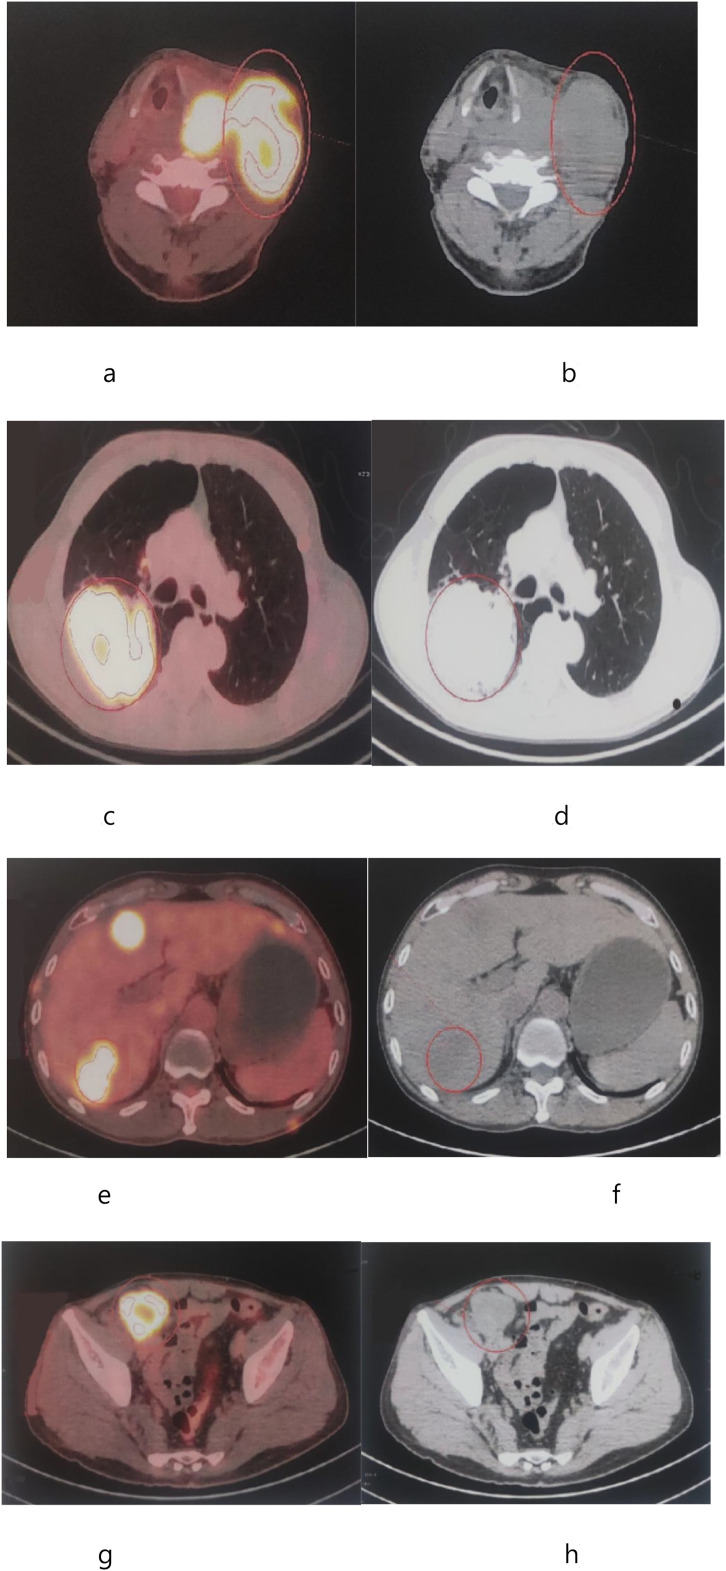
PET/CT images of the patient before treatment. (a, b) PET imaging shows increased [18F]-FDG uptake in the left thyroid (SUVmax 25.8) and lymph node (SUVmax 30). (c, d) PET imaging shows increased [18F]-FDG uptake in the right lung and pleura (SUVmax 20.6). (e, f) PET imaging shows increased [18F]-FDG uptake in the liver (SUVmax 30). (g, h) PET imaging shows increased [18F]-FDG uptake in the pelvic cavity (SUVmax 32).
